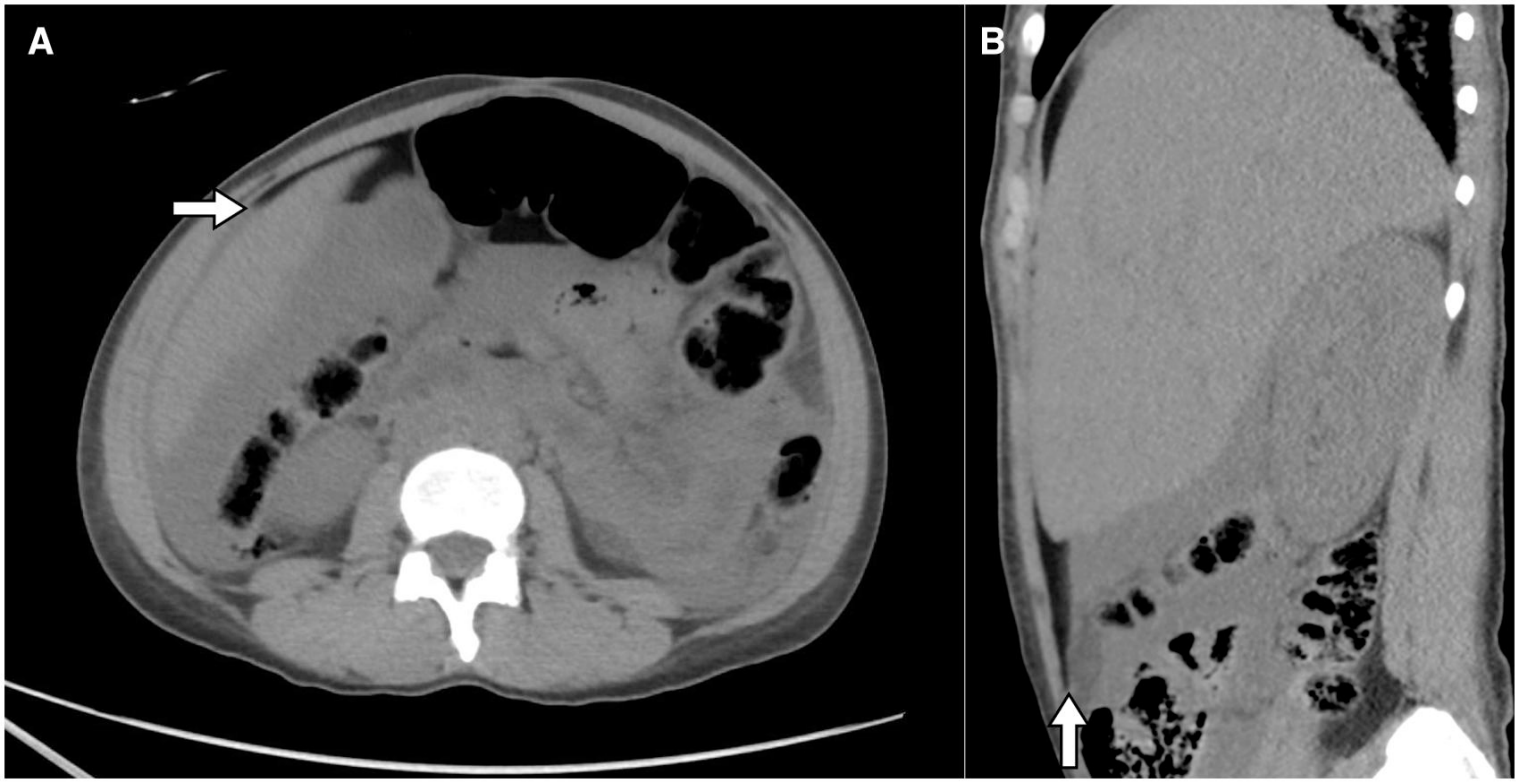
Axial CT section at the level of the upper abdomen (A) and right parasagittal reformation (B) shows fluid attenuation in the dependent areas, and fat attenuation in the non-dependent areas, forming a fat- fluid level. The anterior margin of the liver (arrow in (A)) is surrounded by the fat attenuation fluid.
